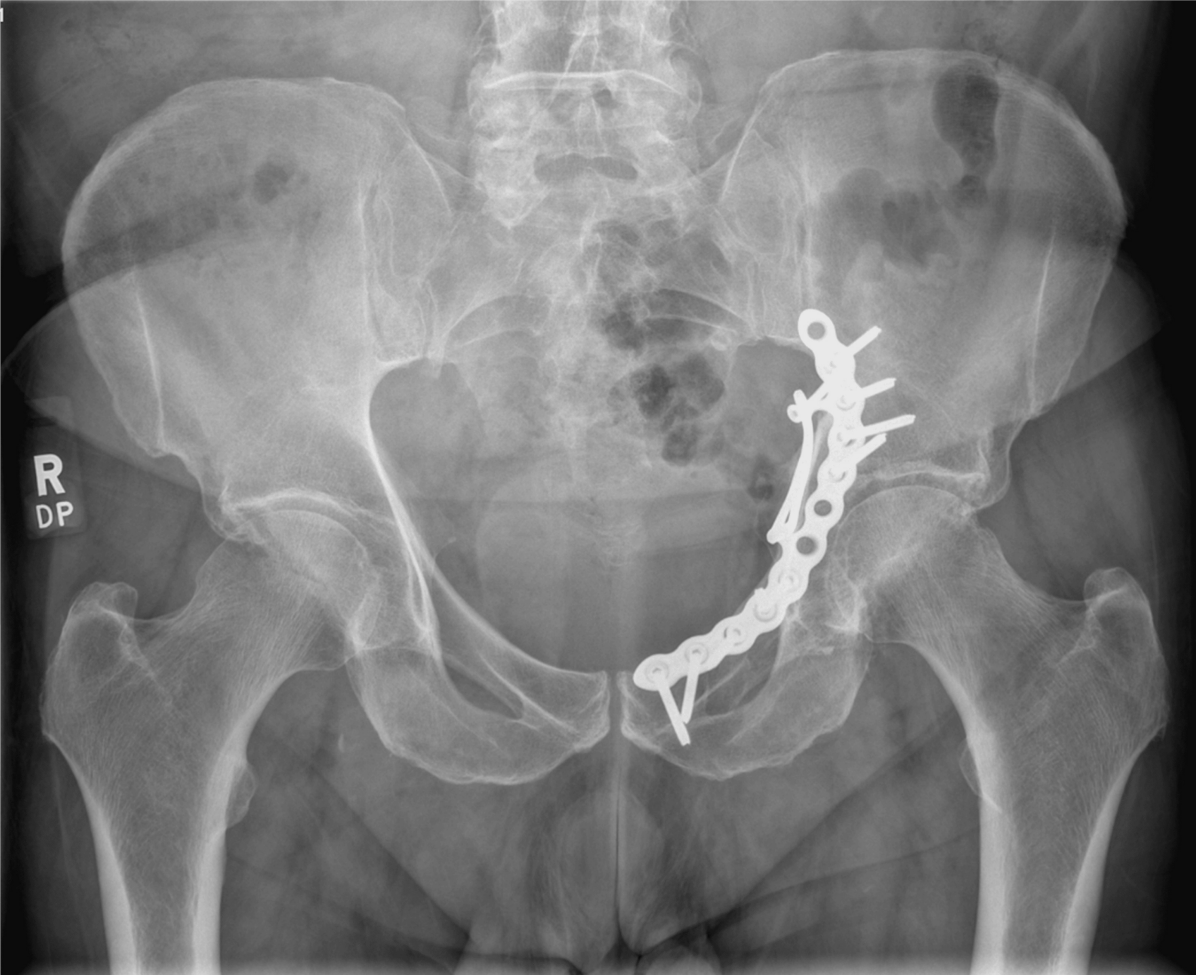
Anteroposterior pelvic radiograph in a patient, five years following fixation of a displaced acetabular fracture using an anatomical suprapectineal plate
